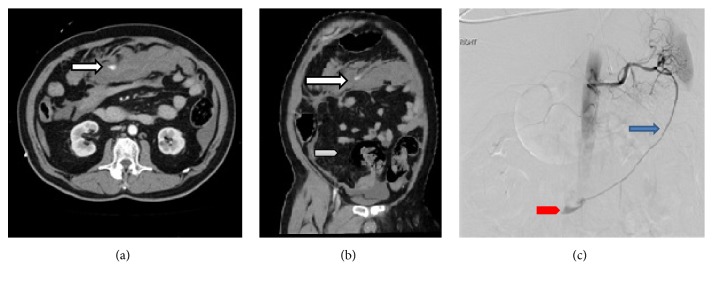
Case 1: Figures 1(a), 1(b), and 1(c). (a) Axial CT scan showing active contrast extravasation in the region of greater omentum. (b) Coronal MIP image showing actively bleeding aberrant artery arising from the splenic artery (arrow) and extending diagonally into the right iliac fossa, supplying the fat containing lesion (arrow head ). (c) Pre-embolization angiogram depicting the aberrant artery (blue arrow ). There is active contrast extravasation inferiorly (red arrow head ).
